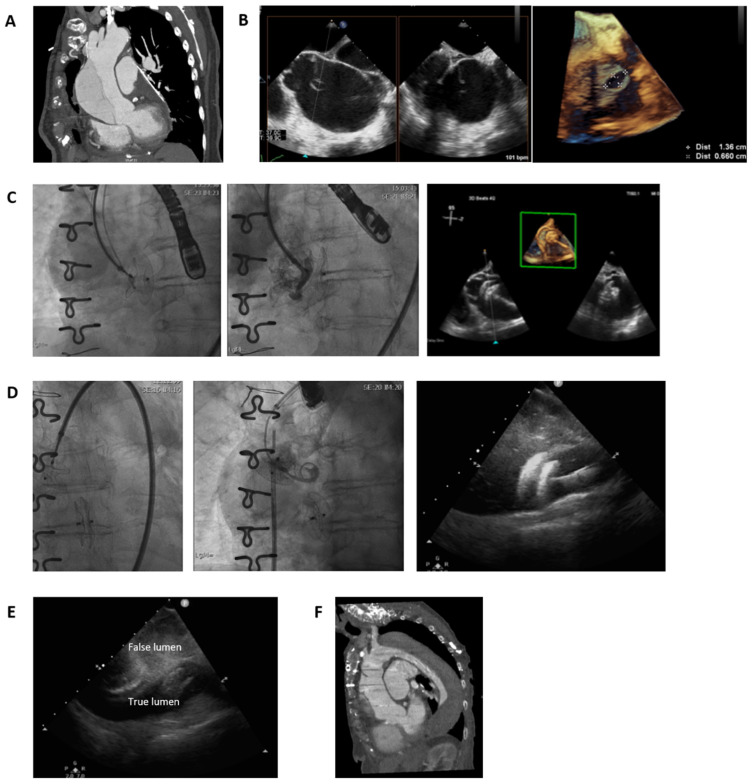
Percutaneous treatment of type A aortic dissection using atrial septal defect occlusion device: (A) Thoracic CT scan demonstrating a type A aortic dissection along the left lateral aspect of the aorta, with coronary arteries and supra-aortic vessels perfused from the true lumen. (B) Proximal entry site of the aortic dissection located 2 cm above the aortic valve, measuring 1.3 × 0.7 cm. (C) Occlusion of the proximal dissection entry site using a self-expandable, double-disc, 20 mm atrial septal defect occlusion, positioned from the false lumen to the true lumen. (D) Occlusion of the residual aortic arch dissection entry site using a self-expandable, double-disc, 18 mm atrial septal defect occlusion device. (E) Intracardiac echocardiography demonstrating precise and stable deployment of both occlusion devices, with immediate stasis observed in the false lumen. (F) Two-year follow-up CT scan showing complete thrombosis of the false lumen.
